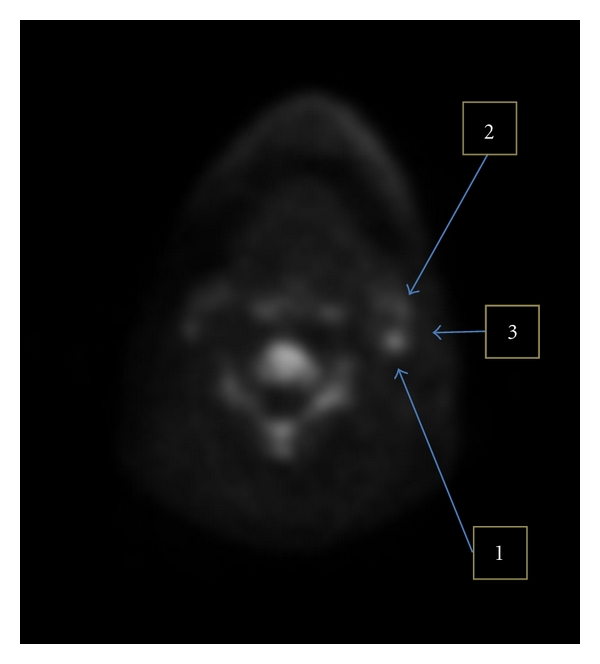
Representative example of target (1) and background definition (2) on SUV image from FLT-PET. The typical background (3) is less indicative of the difficulty associated with delineating the target.
